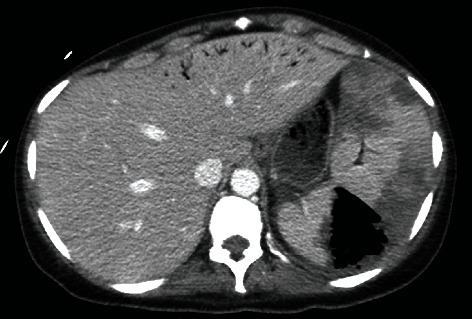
Axial CT image showing a markedly enlarged spleen (20 cm) with multiple areas of hypoattenuation indicating infarction, as well as a large wedge-shaped area of intrasplenic air.
